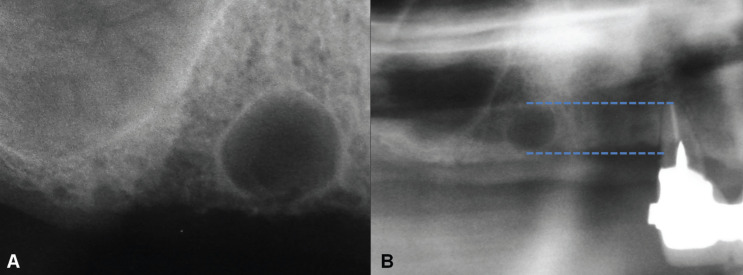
A. Periapical radiograph showing a well-defined rounded unilocular radiolucency in the upper right premolar area. B. Height relationship of the image with the apical third of the upper right lateral incisor.
